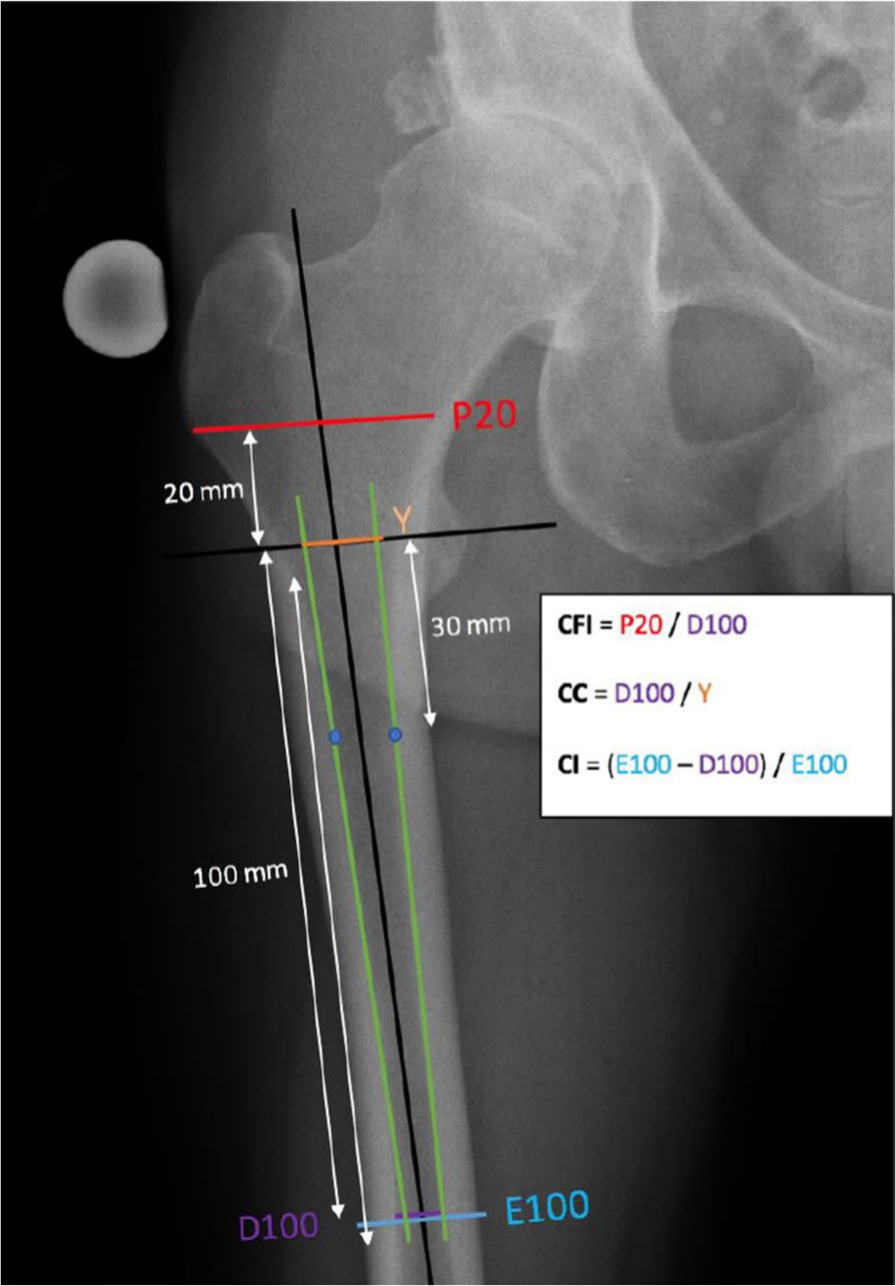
Representation of the measures used on the preoperative radiographs. Preoperative radiograph of a right hip, frontal view CFI = P20/D100; CC = D100/Y; CI = (E100 – D100)/E100; CFI = canal flare index; CC = canal to calcar isthmus; CI = cortical index
